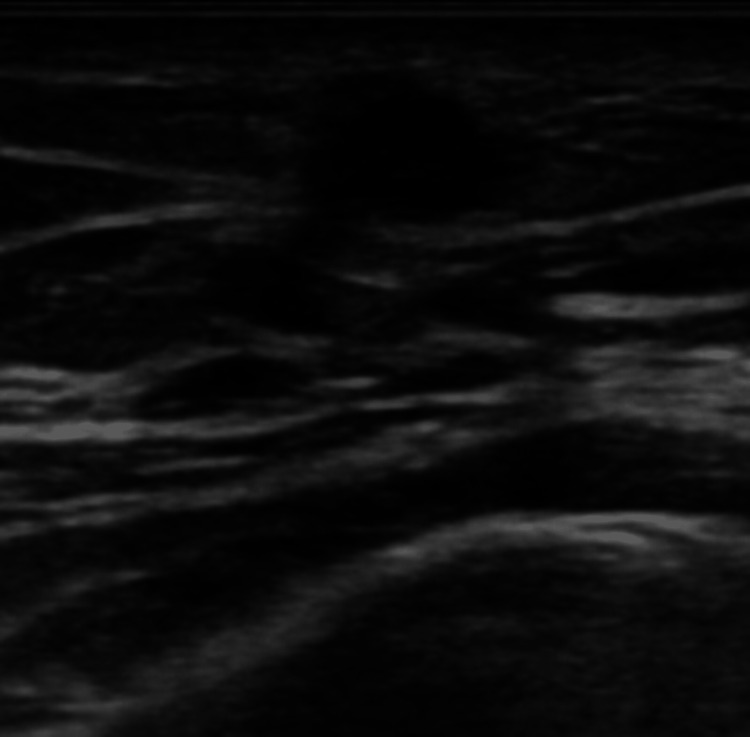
Breast ultrasonography shows an irregular hypoechoic, microlobulated mass parallel to the skin surface, with areas of ill-defined margins and no definite posterior acoustic enhancement or shadowing.
